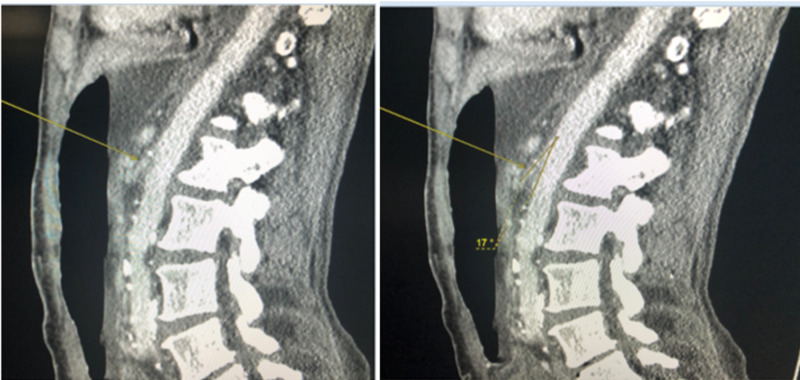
Contrast-enhanced computed tomography of the abdomen showing the origin of the superior mesenteric artery (left side) and an aortomesenteric artery angle of 17 degrees (right side)
